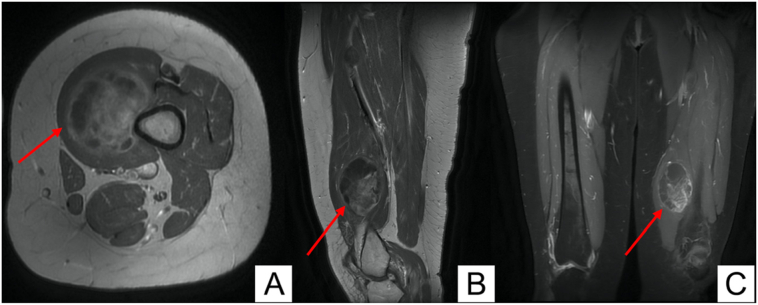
Heterogeneous T1W post-contrast filling in Axial (A), Sagittal (B), and Coronal (C) MRI sections.
